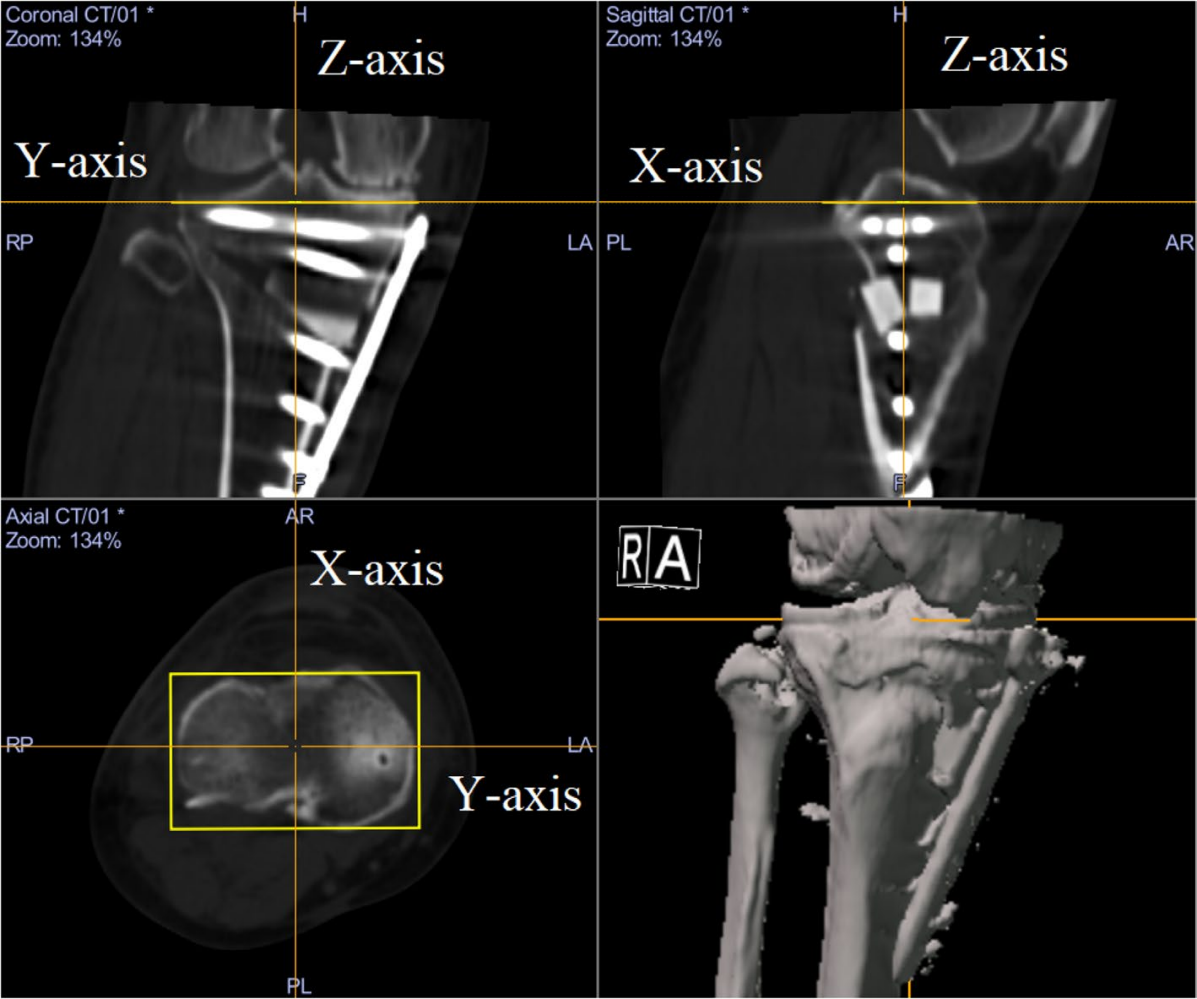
To evaluate the locking plate position on postoperative CT images, the coordinate system was defined around a virtual rectangle fitted onto the contour of the tibial plateau at the level above the proximal end of the fibula parallel to the tibial plateau. Anteroposterior, medial/lateral, and vertical axes was defined as the X-axis, Y-axis and, Z-axis, respectively
